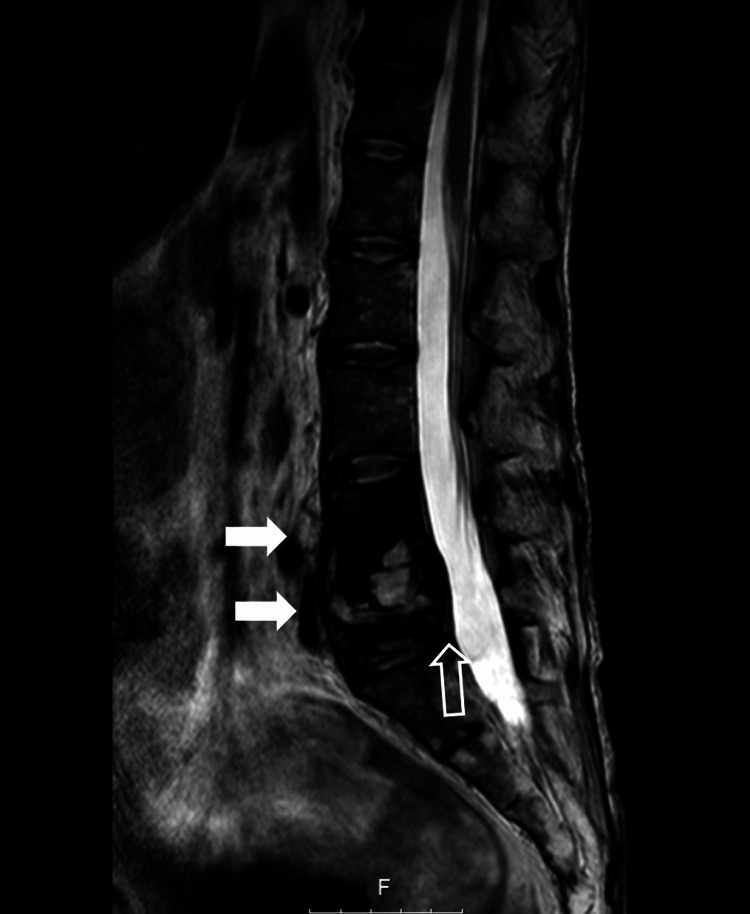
Lumbosacral MRI. Lumbosacral MRI showing posterior displacement of L5 (void arrow) and inflammatory changes in L4, L5, and paravertebral tissues (white arrows).
